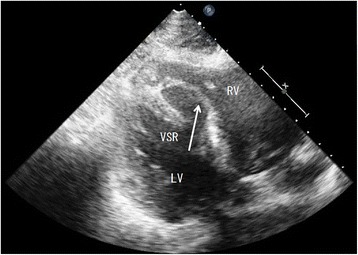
Transthoracic echocardiogram demonstrating the left ventricle (LV), right ventricle (RV), and ventricular septal rupture (VSR)
